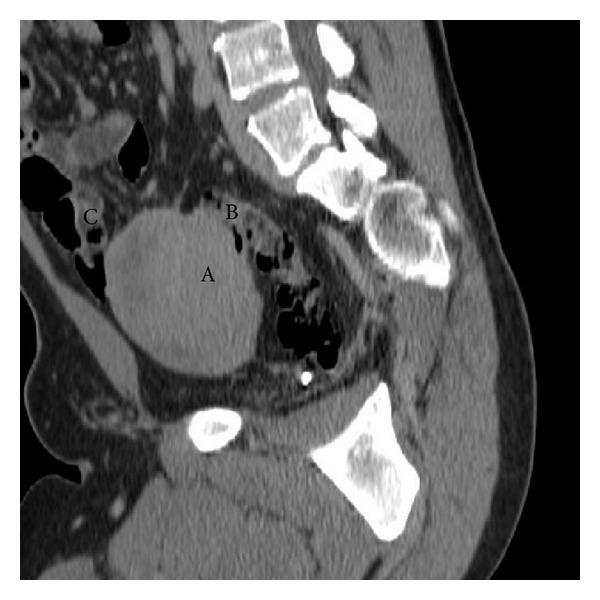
Abdominal computed tomography (sagittal reconstruction): A: desmoid-type fibromatosis; B: sigmoid colon involved; C: ileal loop involved.
